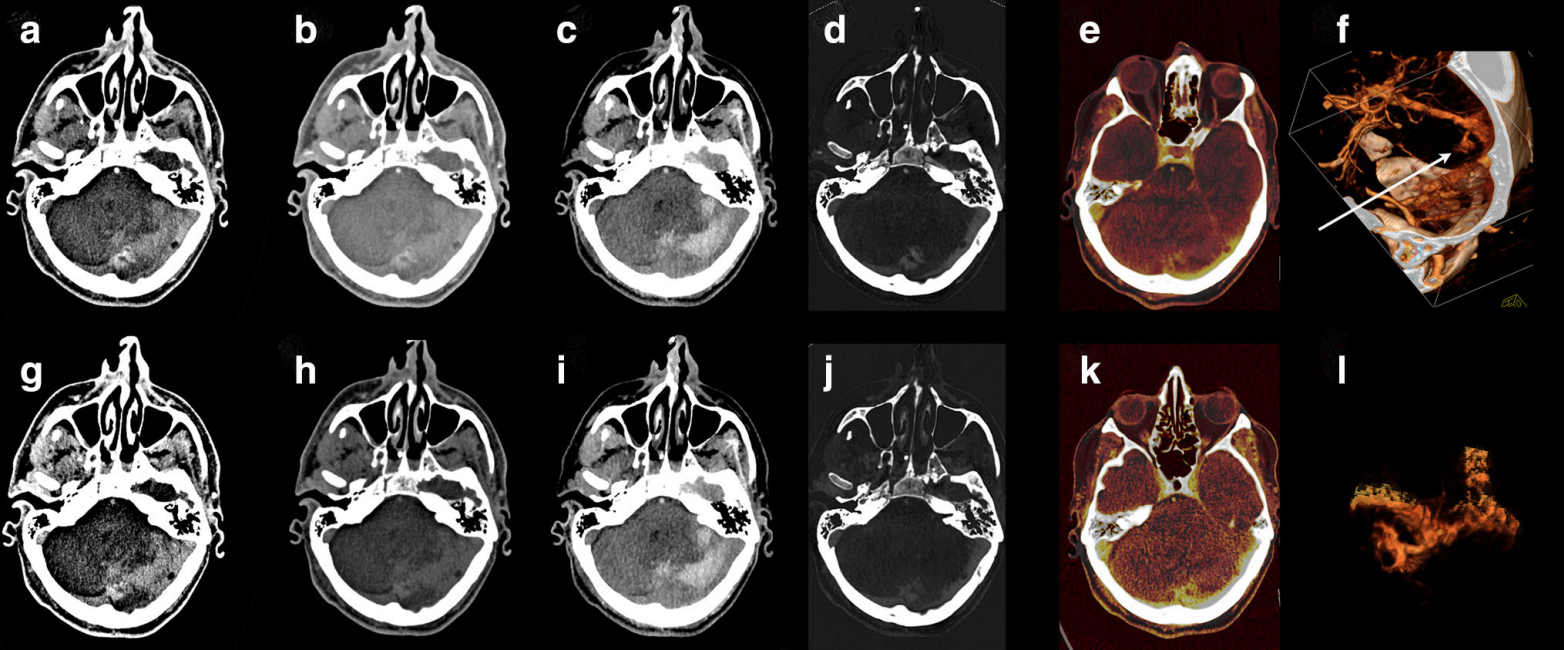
55-year-old male presented with a posterior fossa hemorrhage for which decompression was performed. After surgery DECTA and DECTV were made in search of underlying pathology. Shown are CTA: mixed images with H31 kernel (*a*), mixed images with H20 kernel (*b*), VNC (*c*), IOM (*d*), fusion images (*e*) and manually segmented VR from IOM (*f*, *l*). The lower series shows DECTV: mixed images H31 kernel (*g*), mixed images H20 kernel (*h*), VNC (*i*), IOM (*j*), fusion images (*k*). A residual hematoma is found in the posterior fossa. A developmental venous anomaly (DVA) is shown, best depicted on IOM and fusion images in the arterial phase. VR images from iodine images clearly demonstrate the DVA (*arrow*). This was not visible when VR was done from mixed source images of DECTA/DECTV (not shown) (DECTA 80/Sn140 kVp, 310/155 mAs, CTDI 26.34 mGy, DLP 575 mGycm DECTV 80/Sn140 kVp, 310/155 mAs, CTDI 26.34 mGy, DLP 576 mGycm; 95 cc iodinated contrast 300 mg/ml; injection rate 5 cc/s; 40 cc saline flush 5 cc/s)
